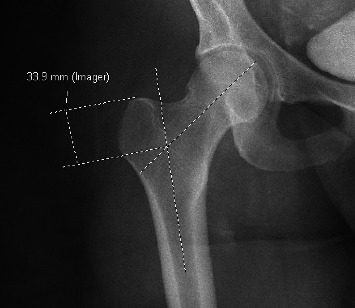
Measurement of GT height via x-ray image in EMR/PACS, with 33.9 mm representing the height of the GT.
